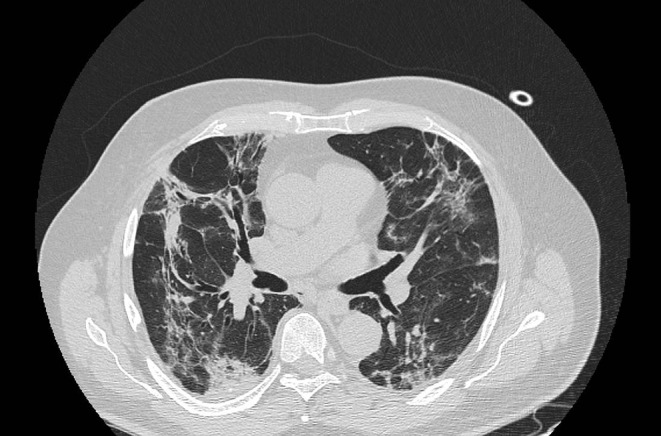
Typical CT findings in patients with COVID-19 pneumonia. Consolidations and GGO, associated with bronchiectasis and crazy paving pattern.
